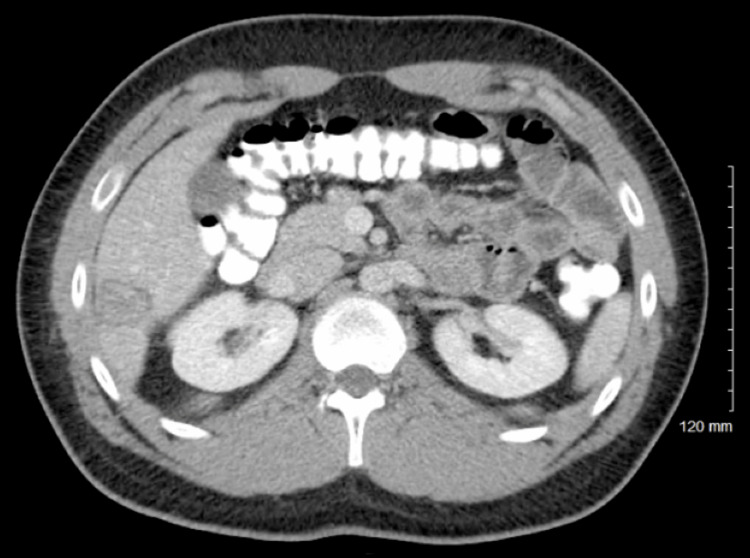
CT after 10 months of chemotherapy and immunotherapy with decreased size of hepatic metastases with the largest measuring 2.9 cm.
